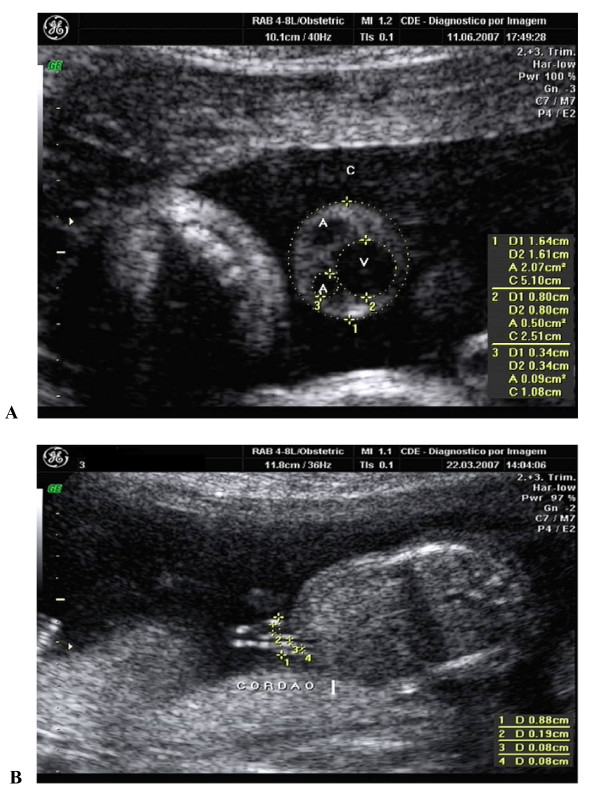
Ultrasonographic measurement A. of the cross-sectional area of the umbilical cord (C), the diameter of the umbilical vein (V) and the umbilical artery (A); and B. of the longitudinal section of the umbilical cord (between 12 and 14 weeks).
